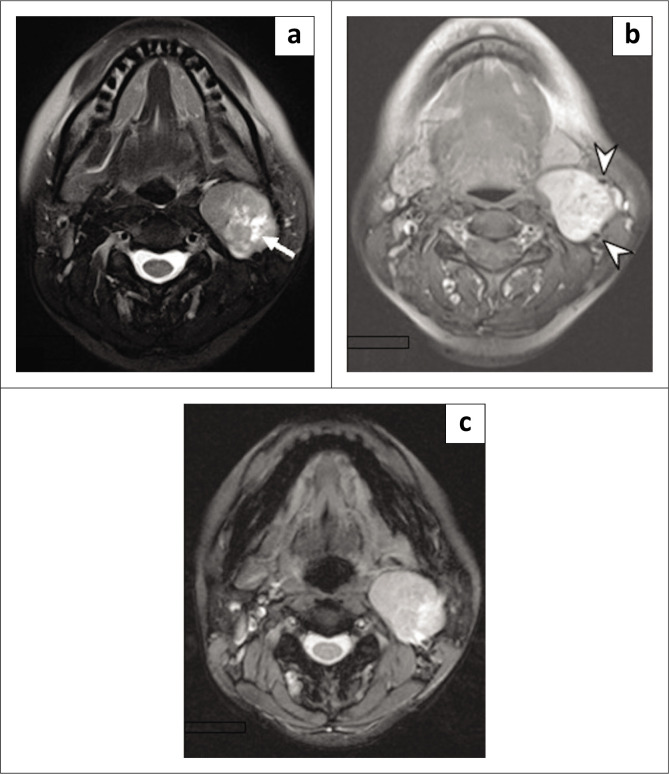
(a) Axial MRI neck T2W fat-saturated image showing a heterogeneously hyperintense soft tissue mass at the left carotid bifurcation with an internal cystic component (white arrow). (b) Axial T1W fat-saturated post-gadolinium image showing splaying of the left internal and external carotid arteries (white arrowheads). (c) Axial FL 2D. Note lack of vascularity within the tumour.
